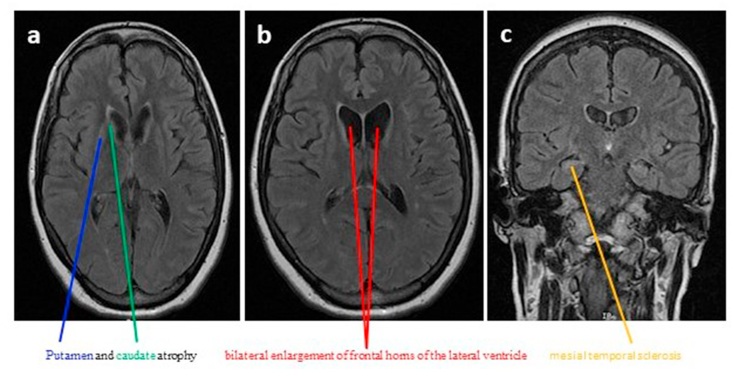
Brain MRI of individual V:5. Axial T2 FLAIR showing bilateral symmetrical caudate and putamen atrophy (a), AXIAL T2 FLAIR showing bilateral enlargement of frontal horns of the lateral ventricle (b), Coronal T2 FLAIR shows hyper-intense signals in both hippocampal regions suggestive of mesial temporal sclerosis (c).
